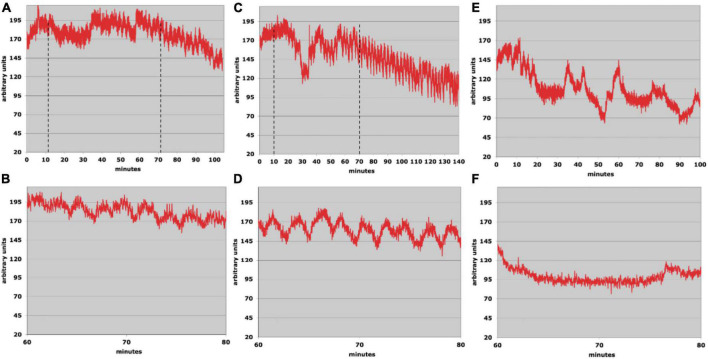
(A,C) Laser Doppler flowmetry data; the dotted lines show the start and stop times of the time-varying caloric vestibular stimulation (tvCVS) stimulation. (B,D) Expanded views of the oscillations from panels (A,C). (E,F) Control run.
